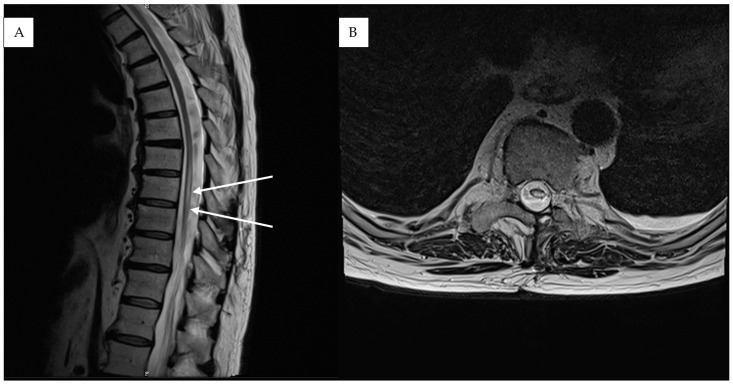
Case results: Post-operative sagittal (A) and axial (B) T2-weighted MR images show decrease in spinal cord edema (white arrows) three months after the operation.
